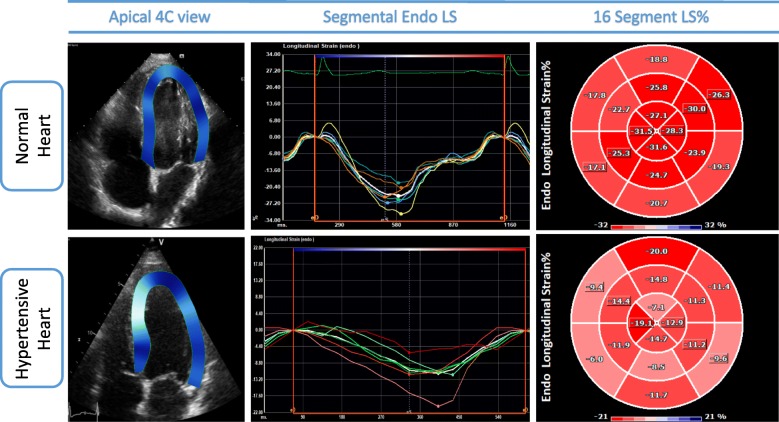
Segmental Speckle Tracking longitudinal strain analysis. Segmental analysis of longitudinal strain in a normal heart (top row) compared to a patient with advanced hypertensive heart disease with reduced longitudinal strain (lower row). The longitudinal strain curves show deformation of individual segments which is reflected in the 16-segment Bull’s eye. In this case, segmental strain is relatively globally impaired due to the homogeneous myocardial distribution of the condition. Abbreviations: Apical 4C, apical four chamber view; Endo, endocardial, LS, longitudinal strain
